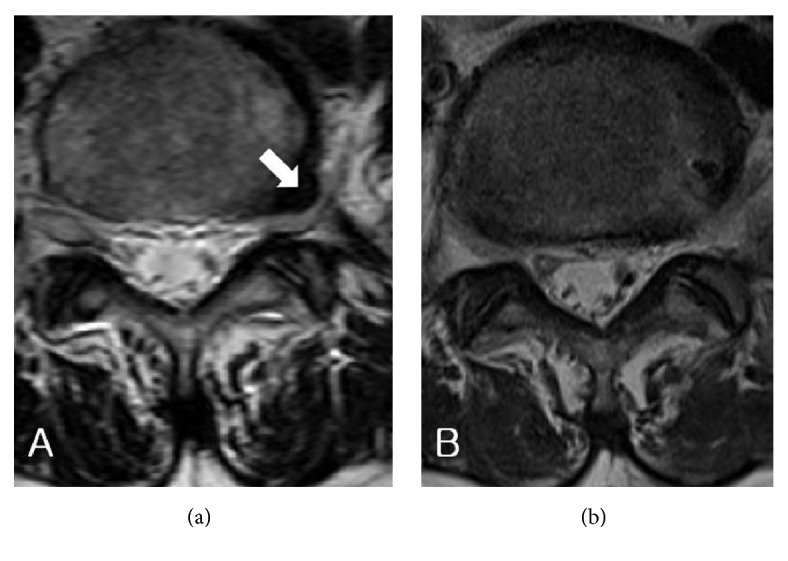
(a) Pre-op axial MRI showing left-side extra-foraminal disc herniation (white arrow) at L5-S1 level. (b) Post-op axial MRI showing removal of extra-foraminal disc herniation.
